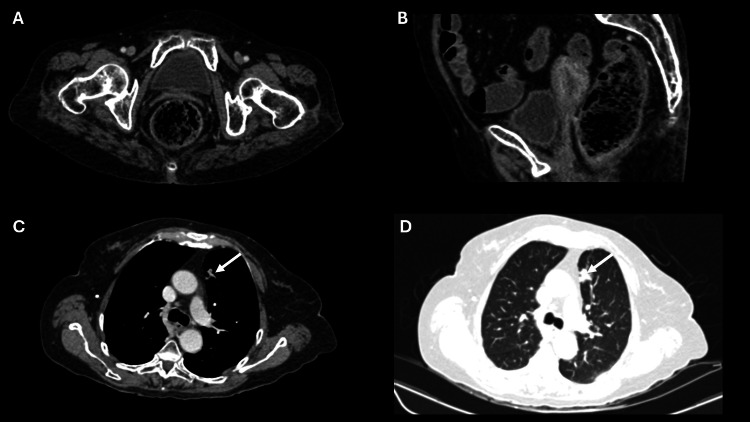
Cross-sectional (A) and sagittal (B) CT images reveal the resolution of the lesion involving the cervix. Cross-sectional images (C & D) reveal a persistent enhancing nodular soft tissue lesion in the anterior segment of the left lung upper lobe (white arrows).
